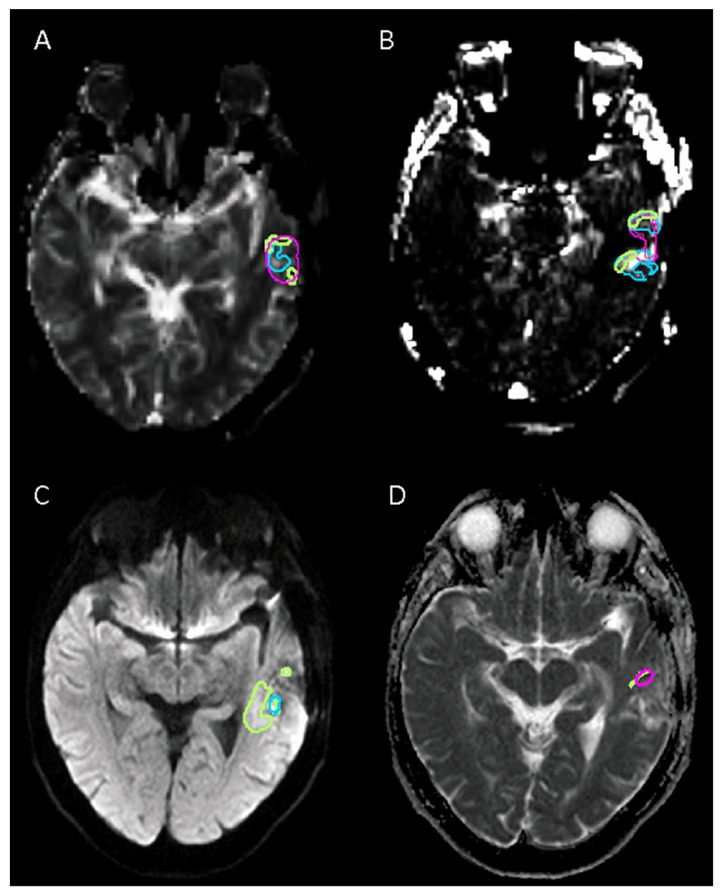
Example of sequences with poor agreement for tumor volume delineation in high-grade glioma between readers: (A) Relative cerebral blood volume (rCVB) corrected for contrast leakage shows. (B) Permeability estimation map (k2). (C) Diffusion-weighted imaging (DWI b1000). (D) Apparent diffusion coefficient (ADC) map. In this case, only two readers delineated tumor volume with an ADC map.
